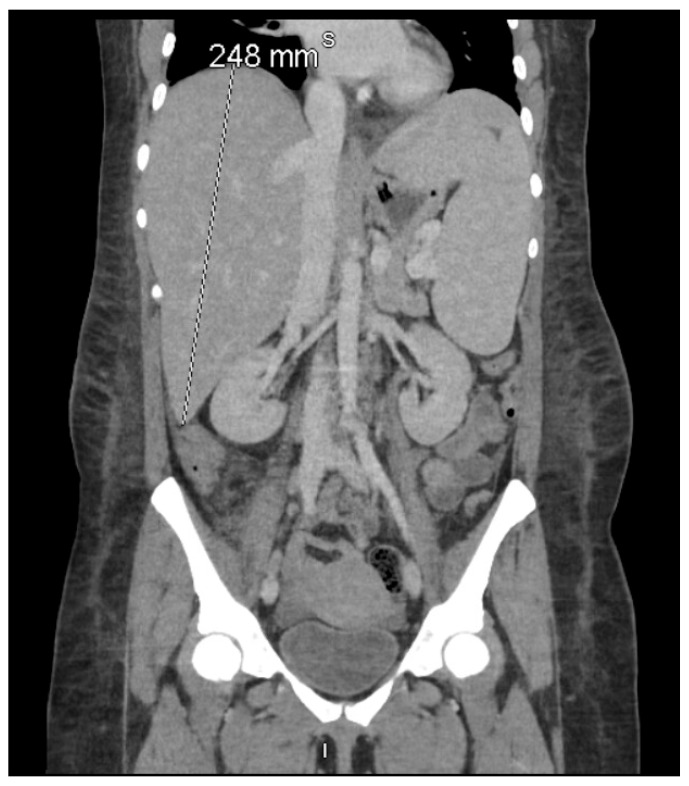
Coronal contrast-enhanced computed tomography (CT) of the abdomen and pelvis demonstrating pronounced hepatosplenomegaly, with the liver measuring 248 mm craniocaudally and the spleen measuring 186 mm in its long axis. According to the HLH-2004 criteria established by the Histiocyte Society, splenomegaly—clinically defined as palpable ≥2 cm below the left costal margin and radiologically as a CT spleen length >12 cm—is one of eight diagnostic features, of which at least five must be fulfilled for HLH diagnosis [6]. This imaging finding, together with extreme hyperferritinemia (16,375 μg/L) and hypertriglyceridemia (1114 mg/dL) on 11–12 December 2024, fulfilled the hepatosplenomegaly criterion in both the HLH-2004 diagnostic criteria [6] and the revised HLH-2024 guidelines [16].
